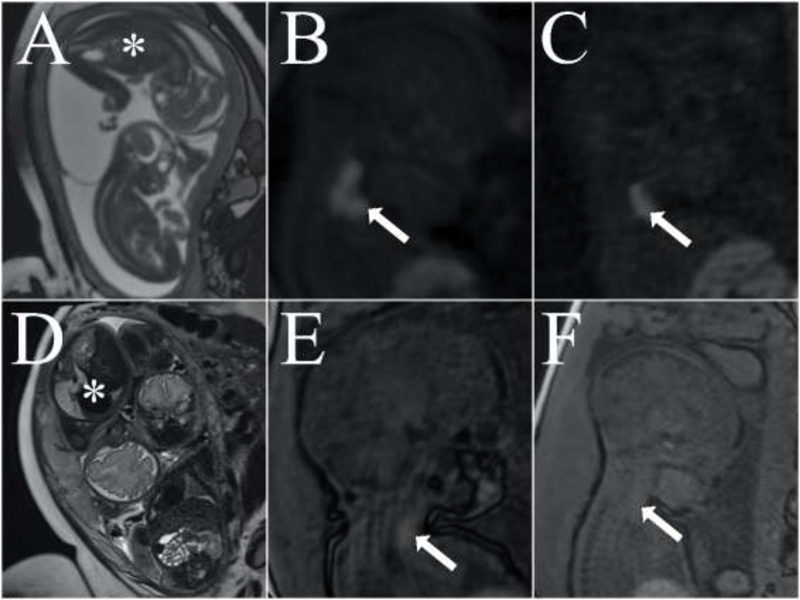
A, B, and C: Magnetic resonance imaging (MRI) at the 28
